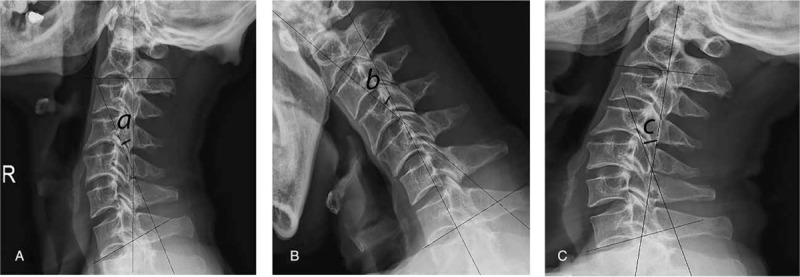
Measurement of C2-C7 Cobb angle (A), and cervical range of motion (B, C) on the lateral cervical spine X-rays. The C2-C7 Cobb angle (A) was defined as the angle of vertical line of the 2 lines, one line parallel to the inferior endplate of the C2 and the other line parallel to the inferior endplate of C7. Cervical range of motion (B, C) = difference of Cobb angle on the flexion and extension view.
